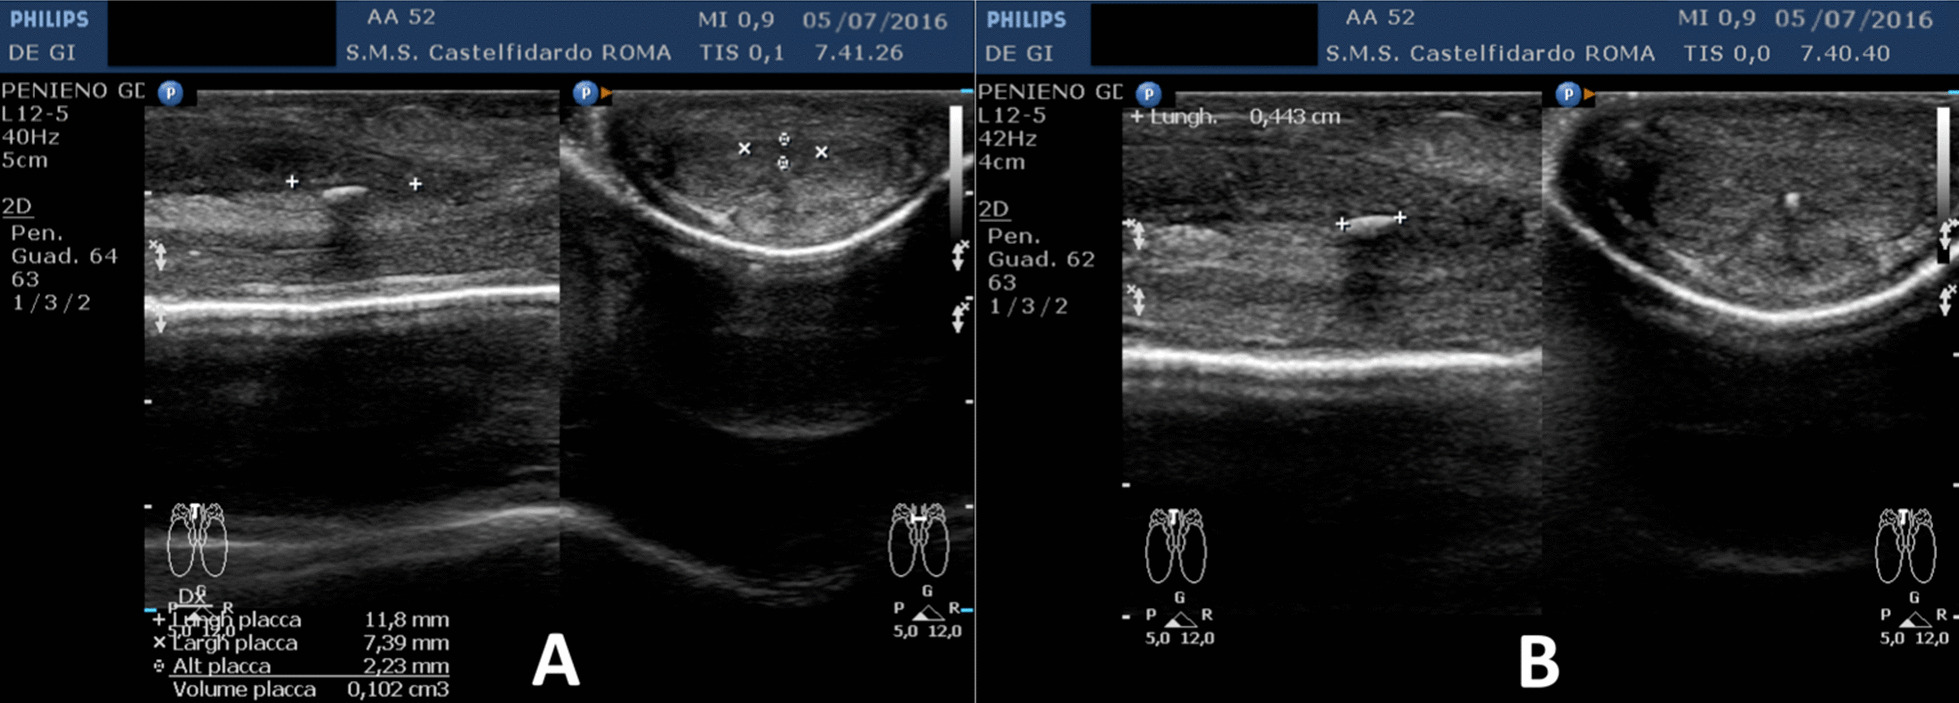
Penile ultrasound after the second treatment cycle (12 months). A Plaque measurement in longitudinal and transverse scan. B Calcification measurement in longitudinal scan
